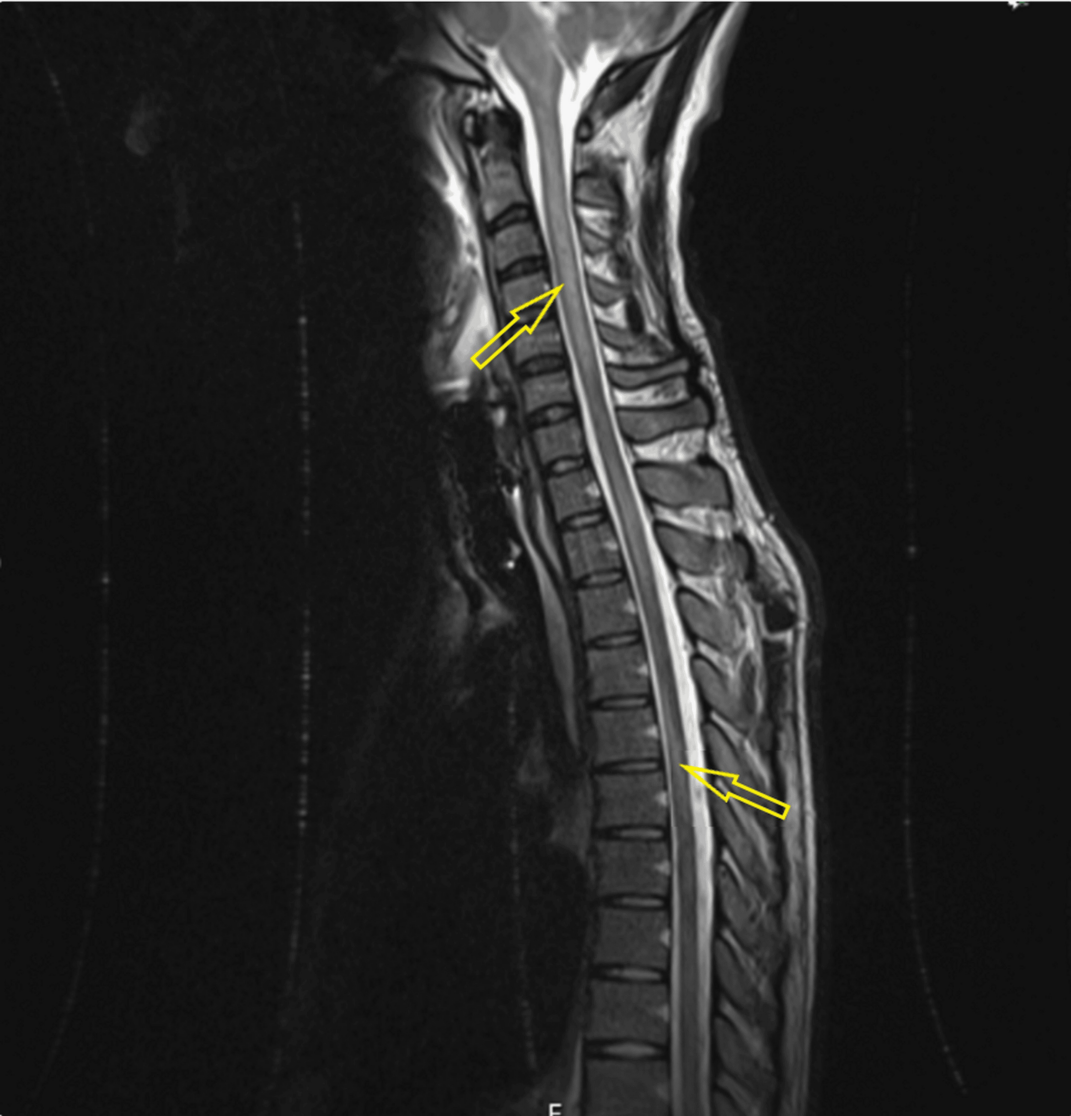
Sagittal T2-weighted MRI showing a long segment of spinal cord inflammation extending from the cervical to thoracic levels (longitudinally extensive transverse myelitis, LETM).
